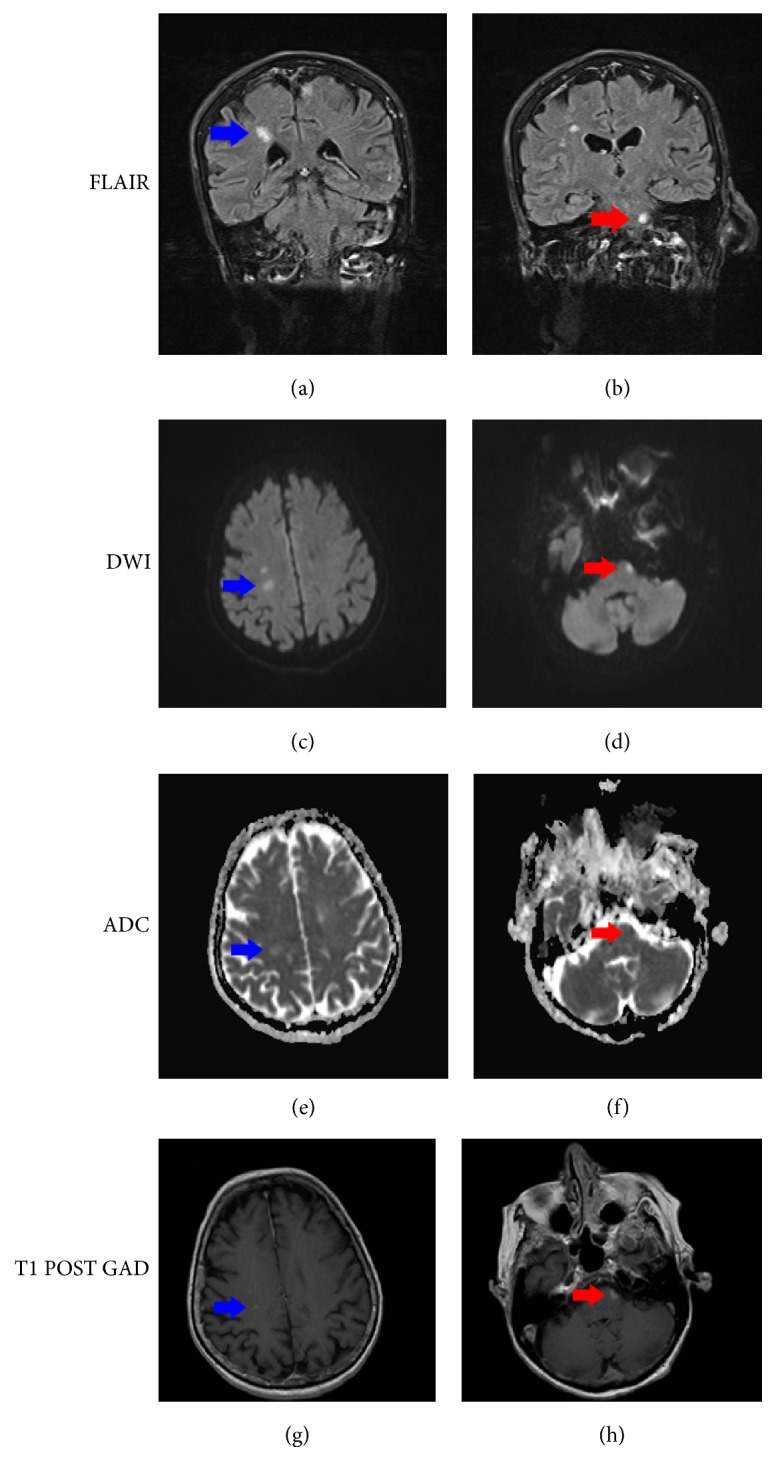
Representative MRI images showing infarcts in the right parietal lobe (blue arrow; images (a), (c), (e), and (g)) and left ventral pons (red arrow; images (b), (d), (f), and (h)). The right parietal lesion shows DWI signal change without corresponding ADC changes, consistent with subacute infarct. The left pontine lesion shows both DWI and ADC signal changes suggesting acute infarct. FLAIR, fluid-attenuated inversion recovery. DWI, diffusion weighted imaging. ADC, apparent diffusion coefficient. T1 POST GAD, T1 postgadolinium.
